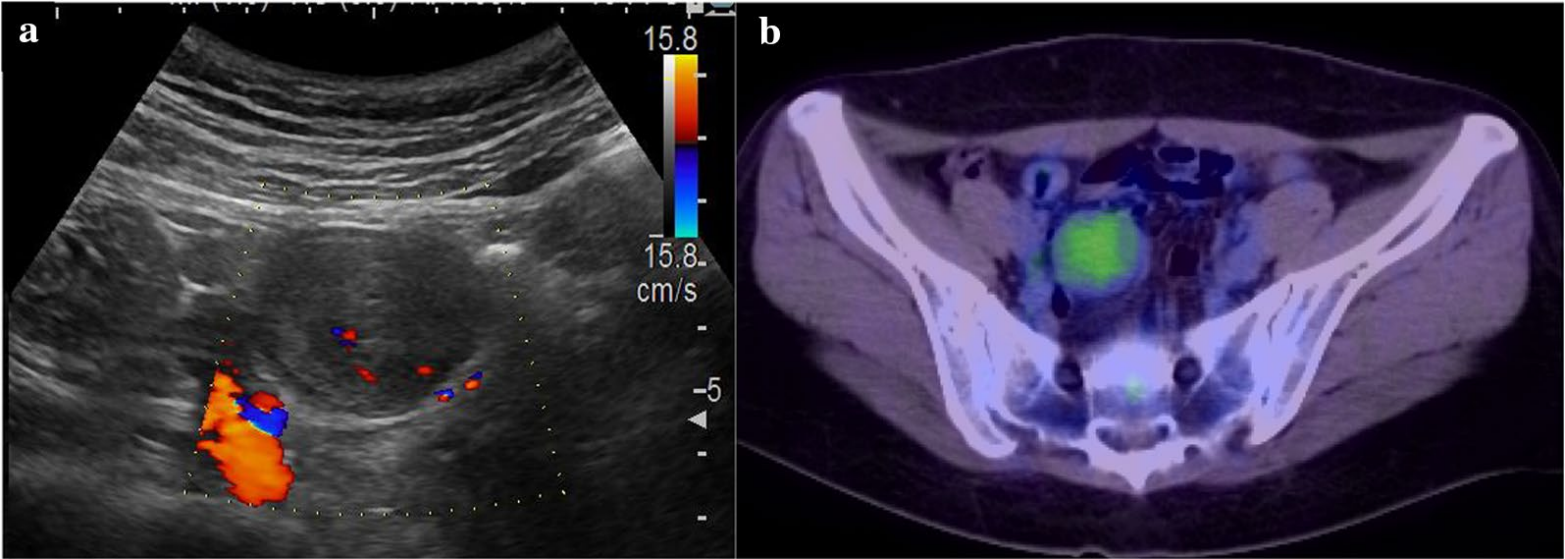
a Abdominal ultrasound image showing heterogeneous hypoechoic mass with a capsule and pulsatile internal blood flow. b Axial FDG PET–CT showing nodular accumulation in the pelvic region, but no distant metastases. *FDG PET–CT* fluorodeoxyglucose positron emission tomography–computed tomography
